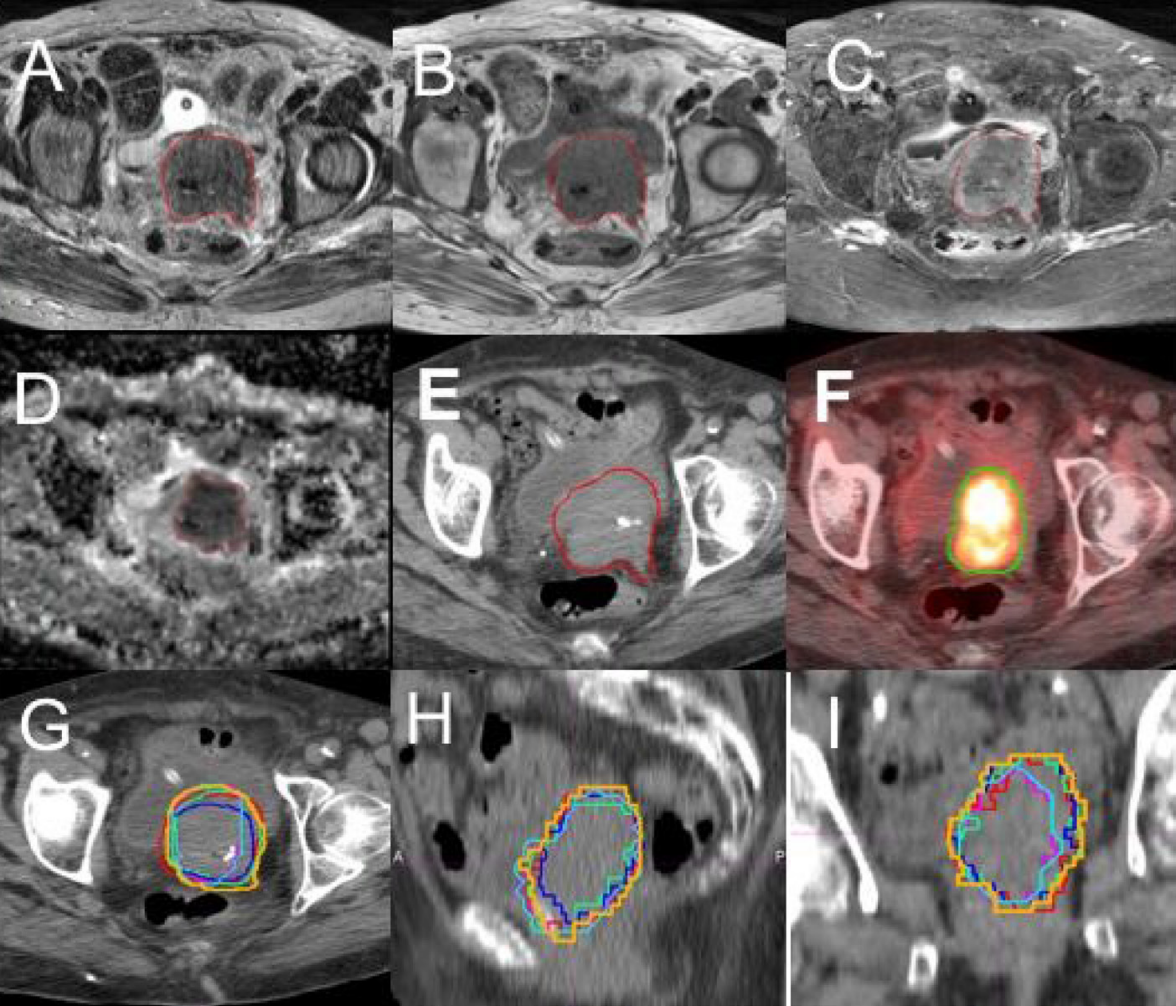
Contours of gross tumor volume (GTV) on multiple imaging modalities for a representative patient (A) T2-weighted MRI; (B) T1-weighted MRI; (C) T1 MRI with fat suppression and contrast (T1F+); (D) apparent diffusion-coefficient (ADC) maps; (E) CT; (F) FDG-PET with SUV = 2.5 threshold. The overlay of the six GTV contours, GTV-T1 (green), GTV-T2 (blue), GTV-T1F+ (red), GTV-ADC (light blue), GTV-SUV_2.5_ (pink) and GTV-CT (brown), on an axial (G), a coronal (H) and a sagittal (I) CT slices are shown.
